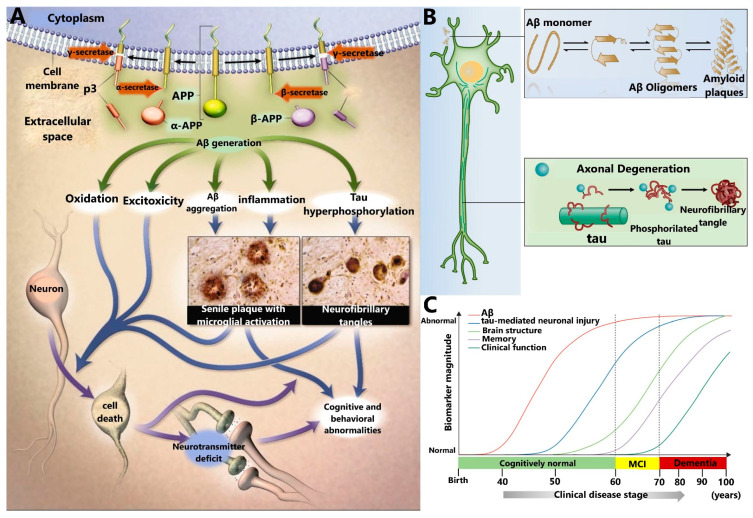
The putative amyloid cascade hypothesis and neuropathological hallmarks of AD. (A) This hypothesis states that AD progresses from the generation of the Aβ peptide from the amyloid precursor protein (APP), through multiple secondary steps, until reaching cell death. From ref. [39] Copyright © 2021 Massachusetts Medical Society. Reprinted with permission from Massachusetts Medical Society. (B) In the amyloid cascade hypothesis, Aβ monomers exist in equilibrium with aggregates of higher molecular mass (dimers, trimers, and oligomers), which finally form a deposit of amyloid plaques at the extracellular level. On the other hand, detachment of P-tau from axonal microtubules would destabilize them, resulting in axonal degeneration at the intracellular level and forming the neurofibrillary tangles (NFTs) at the intracellular level. (C) Hypothetical model showing the time-dependent changes in the levels of AD biomarkers. Levels of Aβ and tau in CSF are identified by PET (positron-emission tomography) and fluorodeoxyglucose-PET. Brain structure is visualized using structural magnetic resonance imaging. MCI: mild cognitive impairment. Republished with permission of Royal Society of Chemistry from ref. [40].
